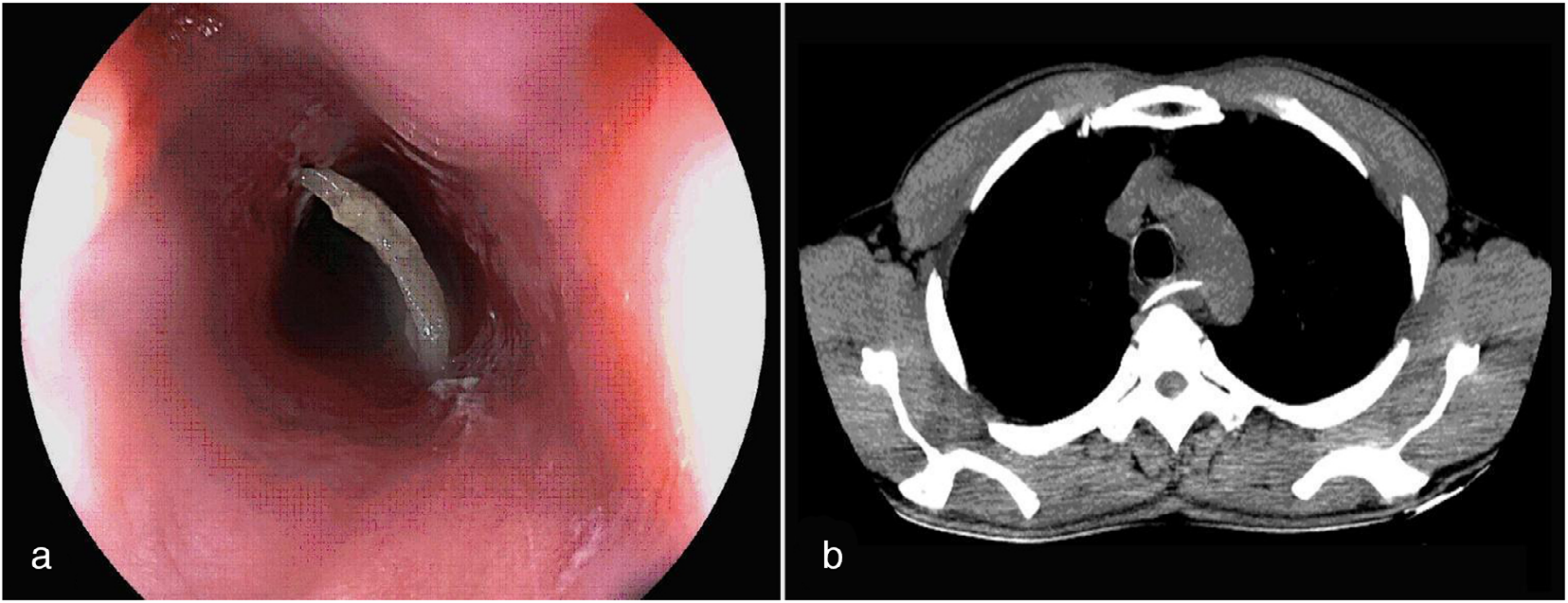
(a) The endoscopic image shows a fish bone foreign body with two ends of a fish bone plugged into the esophageal wall causing inflammation of the mucosa. (b) Chest computed tomography image shows a fish bone foreign body inside the esophagus with one end impinging on the wall of the aorta without perforation; in addition, inflammation with exudative changes around the esophagus.
